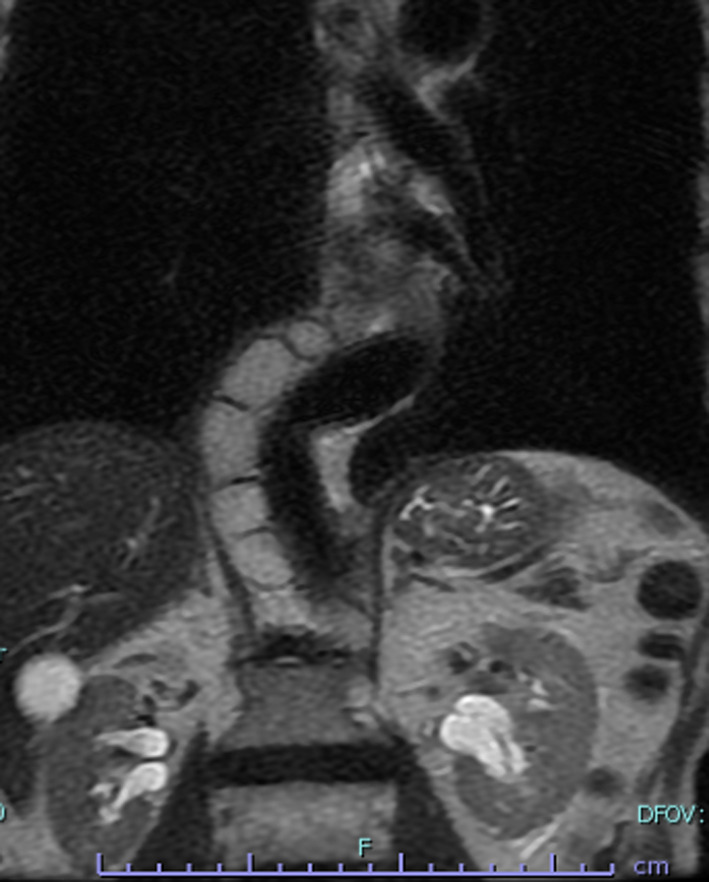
Magnetic resonance imaging showing high intensity on T2-weighted image
